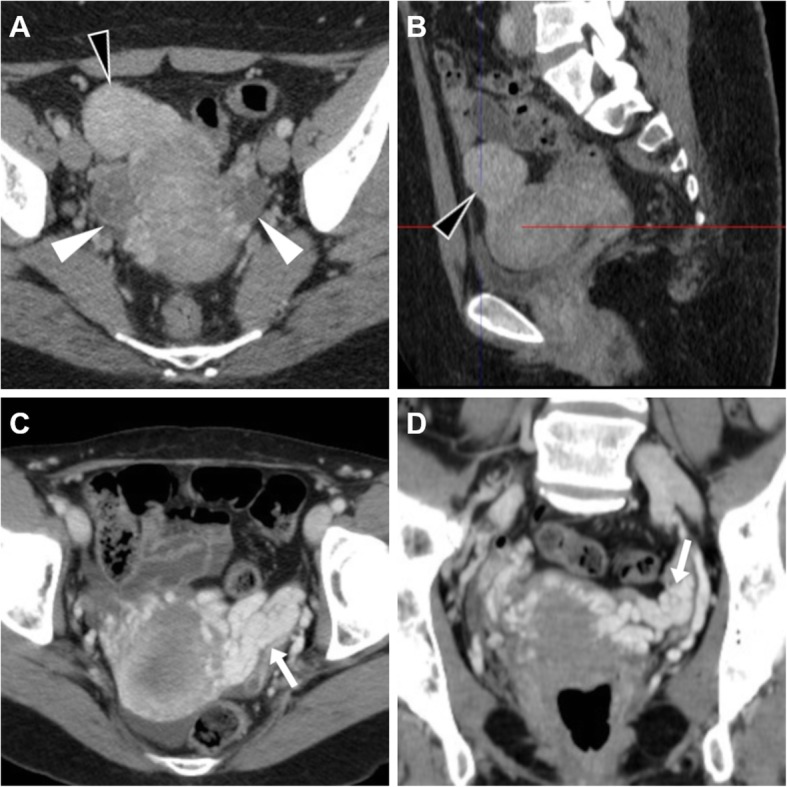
Pelvic congestion syndrome. Axial (a) and coronal (b) CT images showing asymmetric dilatation of the pelvic venous plexus (arrows) reaching 6 mm maximum calibre on the left side
